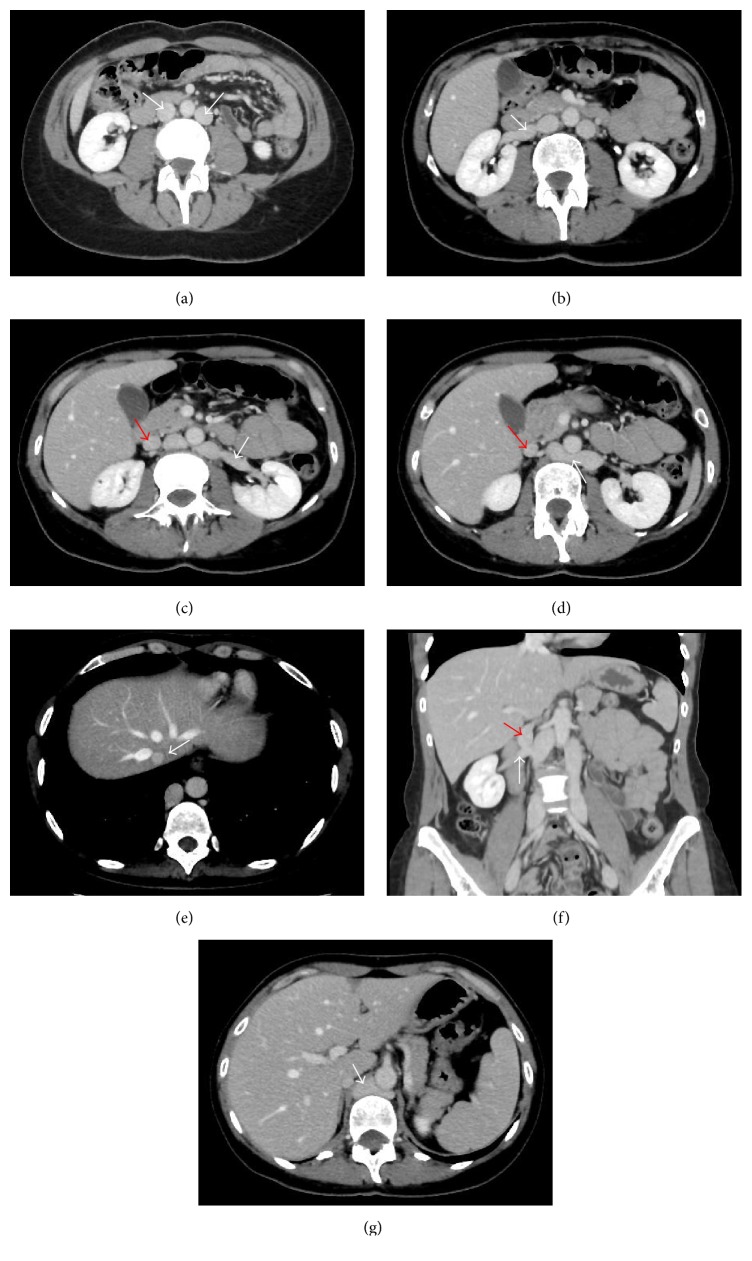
(a) CT axial images that presented from caudal to cranial sections show double IVC (arrows) below the renal veins. (b) The right IVC receives the right renal vein (arrow). (c) The left IVC receives the left renal vein (white arrow); presence of the hepatic portion (red arrow) of the IVC in the CT section before draining into the right renal vein. (d) The left renal vein (white arrow) crosses posterior to the aorta to join the right IVC; continuation of hepatic segment (red arrow). (e) The hepatic veins drain into a hypoplastic hepatic segment (arrow) of the IVC. (f) Coronal MPR image shows the hypoplastic hepatic segment (red arrow) draining into the right renal vein (white arrow), at the confluence with the right IVC. (g) The right IVC continues cephalad as the azygos vein (arrow) within the retrocrural space.
